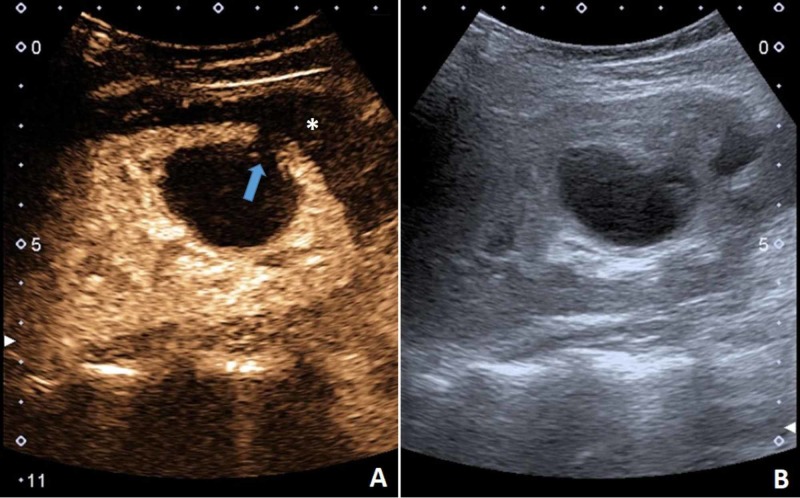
CEUS (A) demonstrating the larger cyst and its communication (arrow) with the subcapsular fluid collection (asterisk). (B) B-mode US. CEUS, contrast-enhanced ultrasound US, ultrasound
